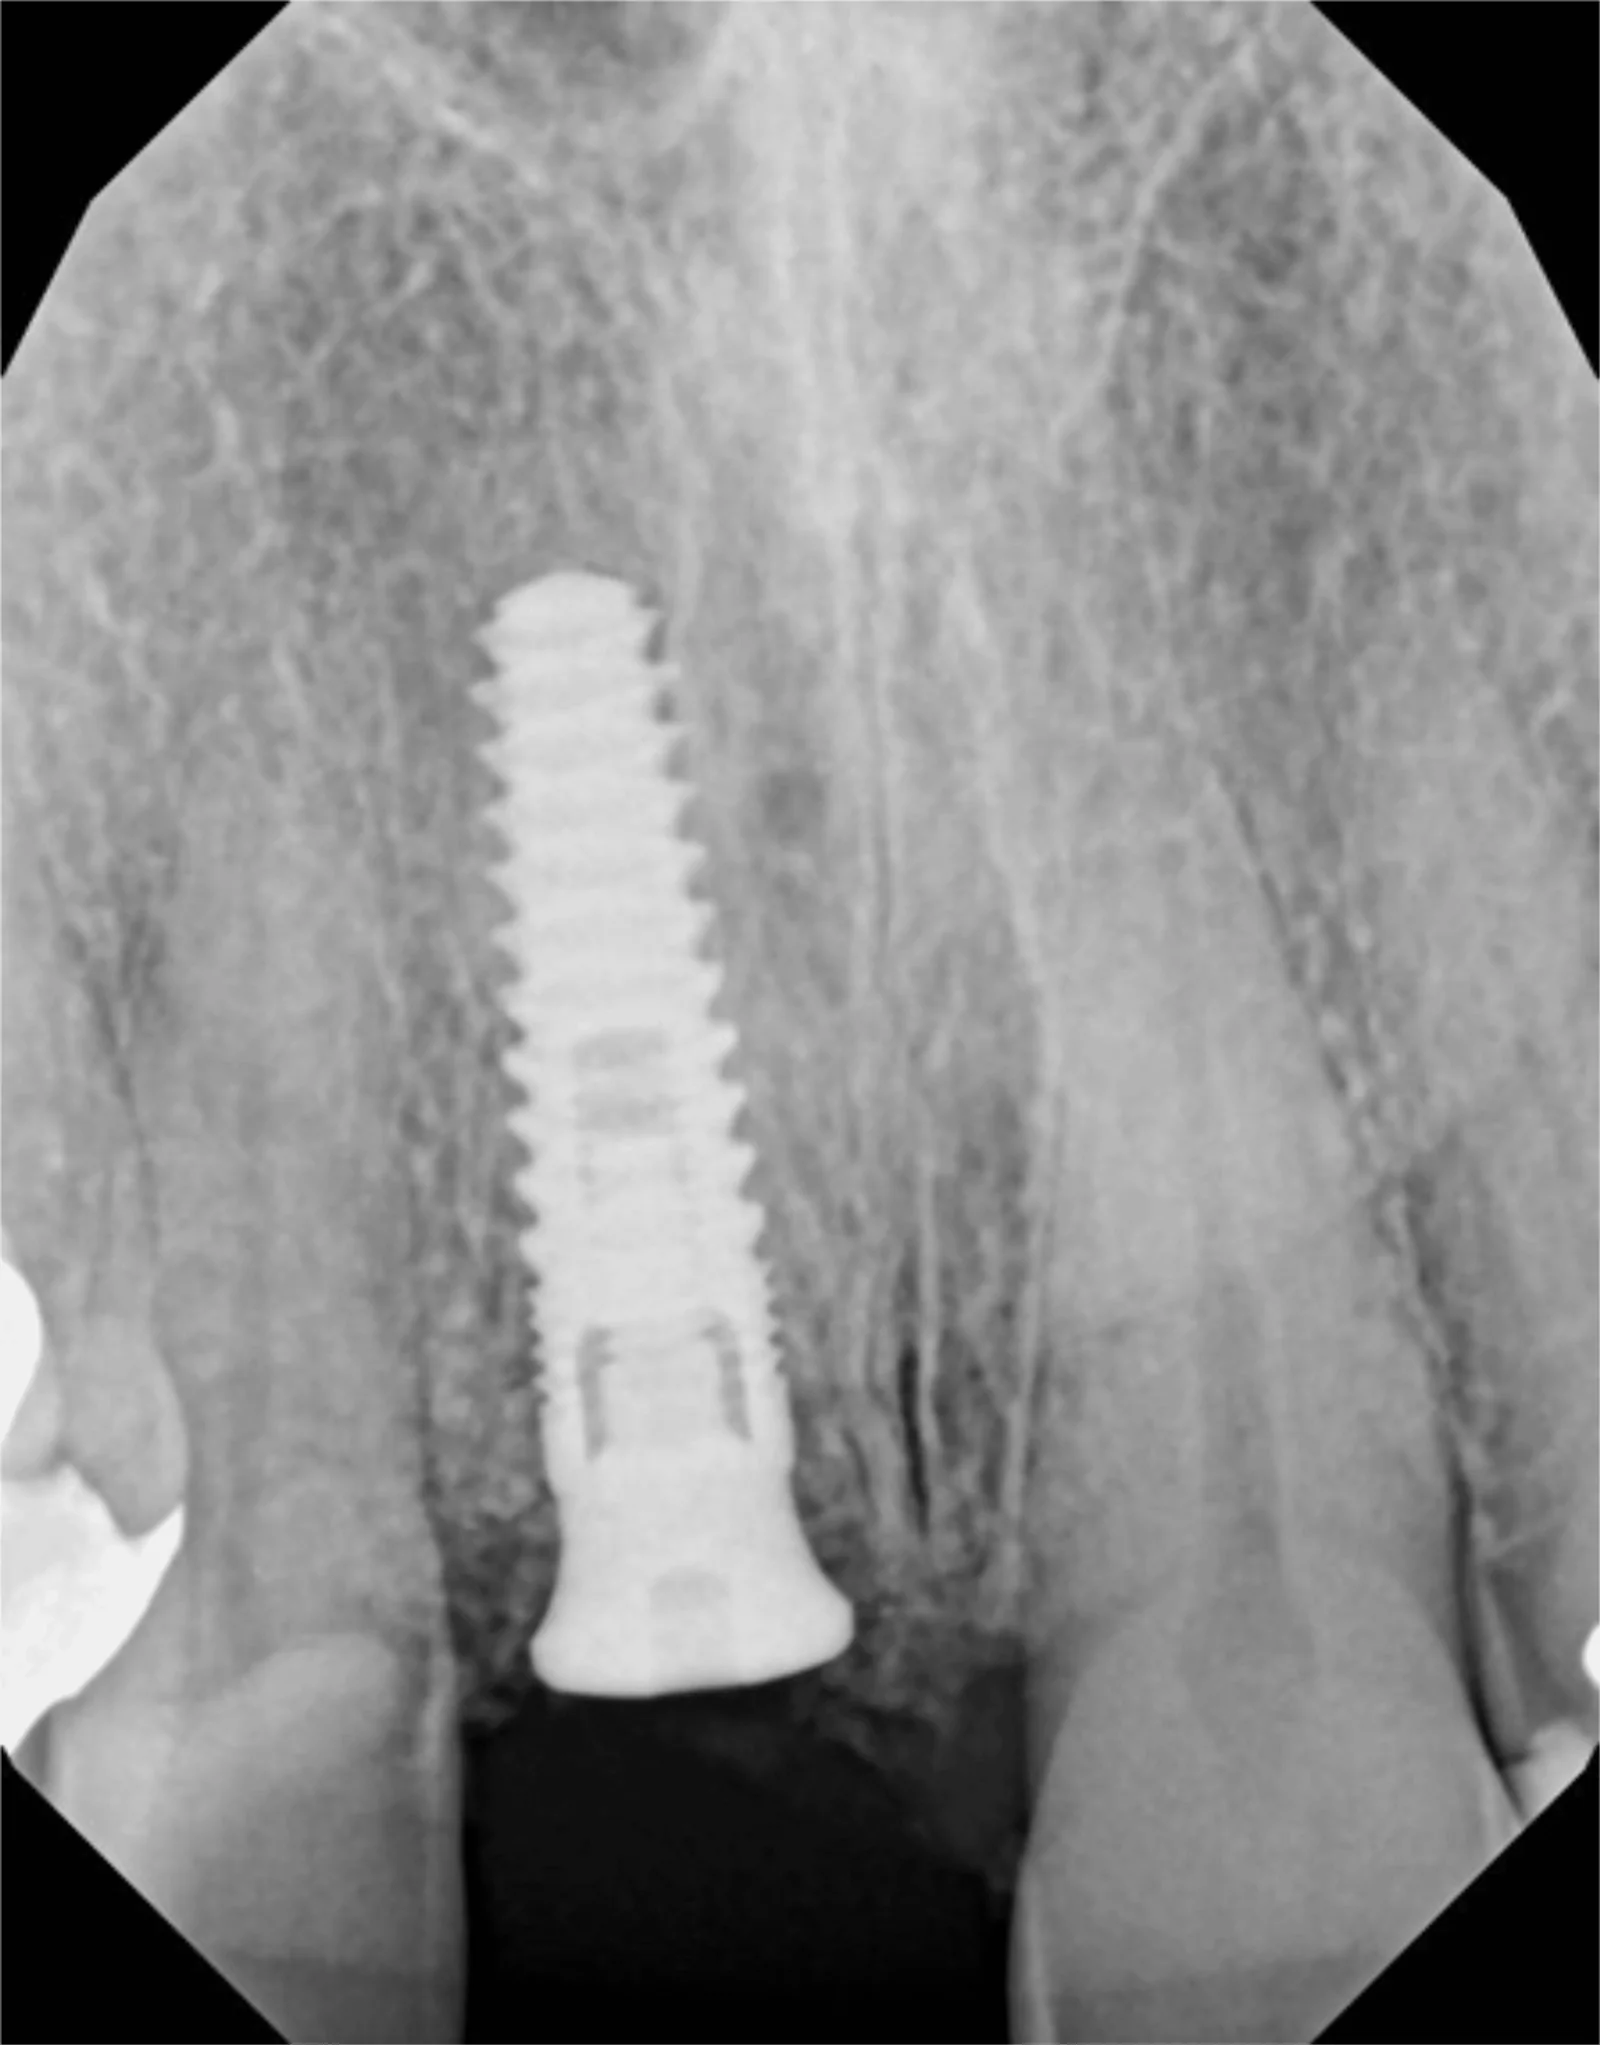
Conventional two-dimensional periapical radiograph of a maxillary central incisor implant following placement. Although the image confirms implant position relative to the adjacent teeth and crestal bone, it cannot accurately assess the buccolingual angulation of the implant within the premaxilla or the thickness of the facial cortical plate. These inherent limitations of two-dimensional imaging may mask facial bone deficiencies, dehiscence, or fenestration that are only reliably detected with three-dimensional imaging.
